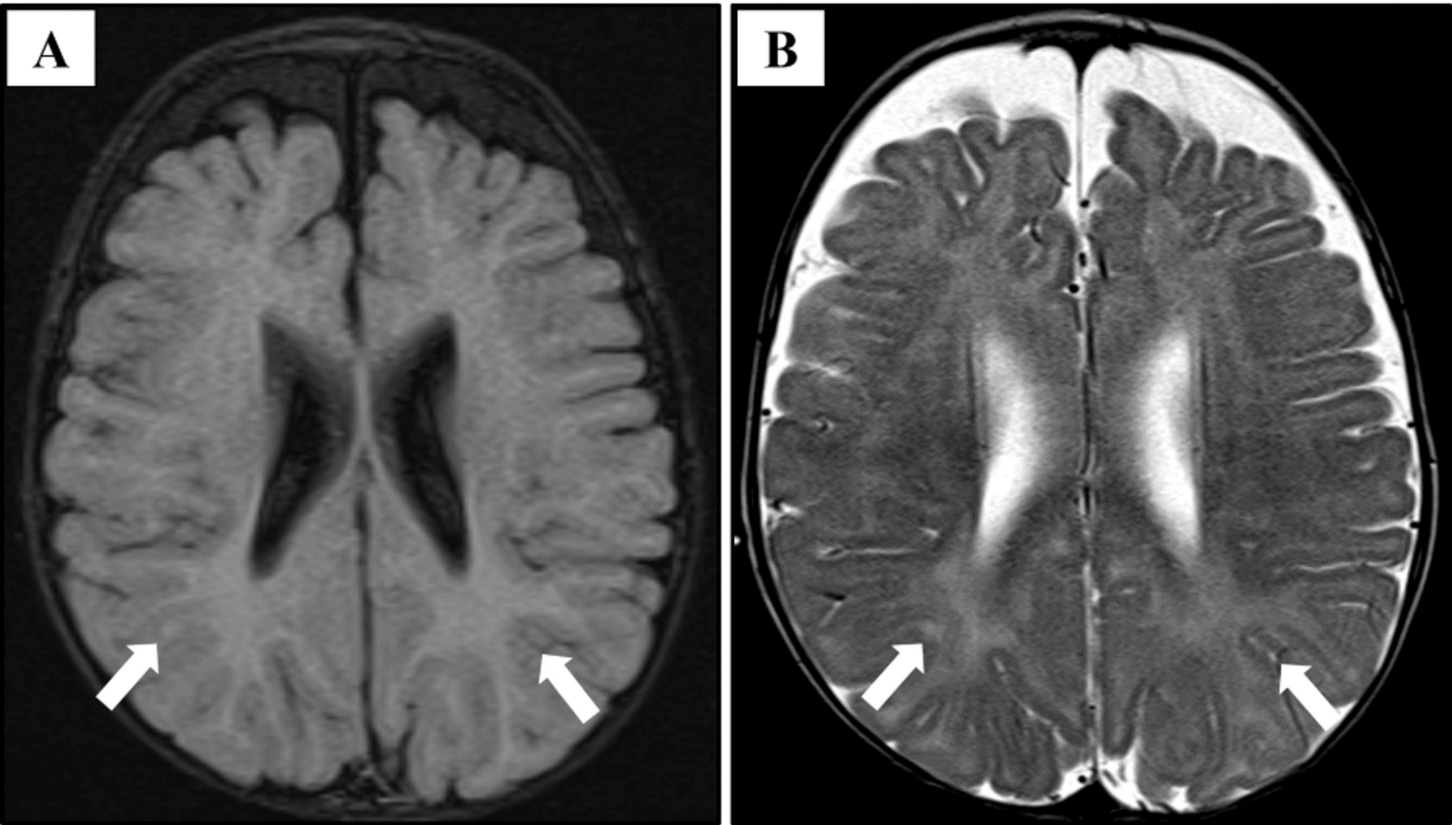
An axial brain MRI of a patient with trichohepatoenteric syndrome (Patient 2) MRI: magnetic resonance imaging (A) A T2-fluid-attenuated inversion recovery (FLAIR) MRI image showing periventricular and peritrigonal white matter hyperintensities extending to some areas of the ventricular ependymal surface (arrows). (B) A T2-weighted MRI image showing the same findings (arrows)
